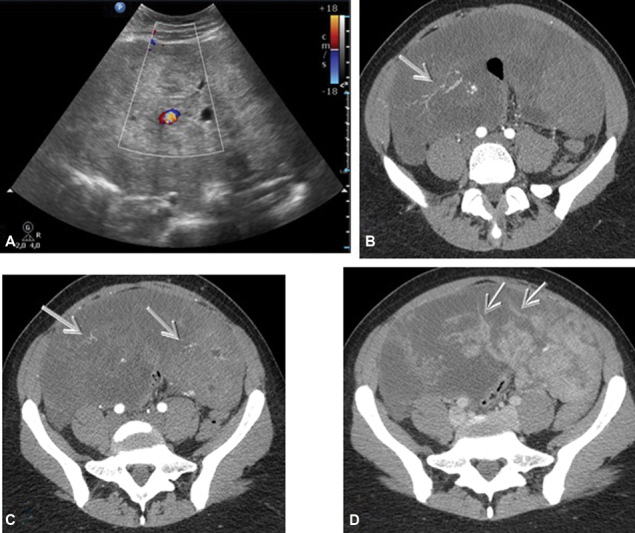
Mass shows internal vascularity on ultrasound color Doppler (A) and axial computed tomography during arterial phase (B-C) (→). Enhancing on venous/portal phase of septa and pseudosepta (D) (→).
